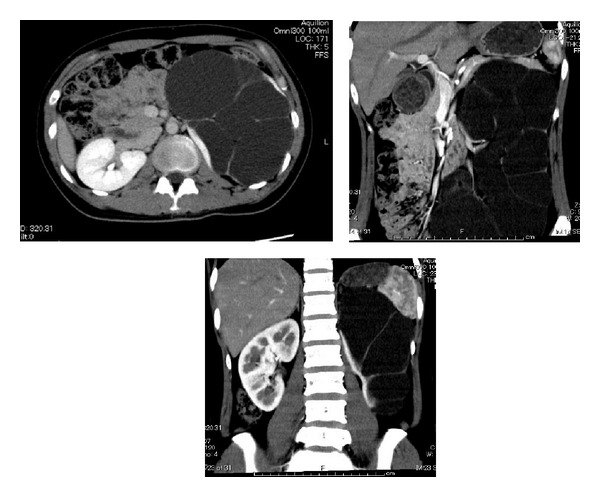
Computed tomography shows dilated left urinary tract and thin renal parenchyma.
